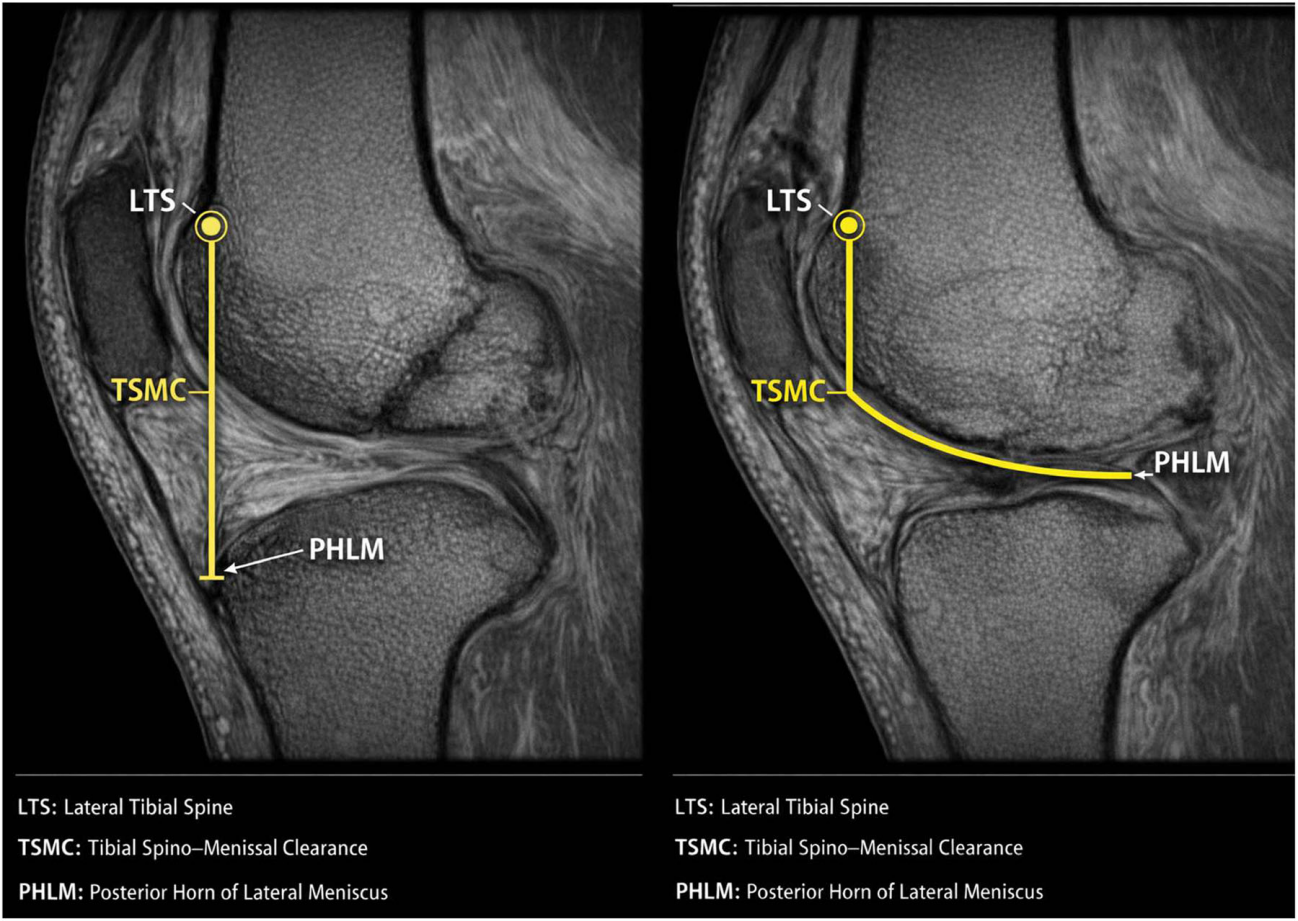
Measurement of TSMC. Sagittal proton density–weighted MRI demonstrating the measurement of TSMC. The sagittal slice showing maximal prominence of the lateral tibial spine is selected. A perpendicular line is drawn from the apex of the lateral tibial spine to the inner margin of the posterior horn of the lateral meniscus. The shortest linear distance between these landmarks is recorded as TSMC. LTS, lateral tibial spine; MRI, magnetic resonance imaging; PHLM, posterior horn of lateral meniscus; TSMC, tibial spino–meniscal clearance.
